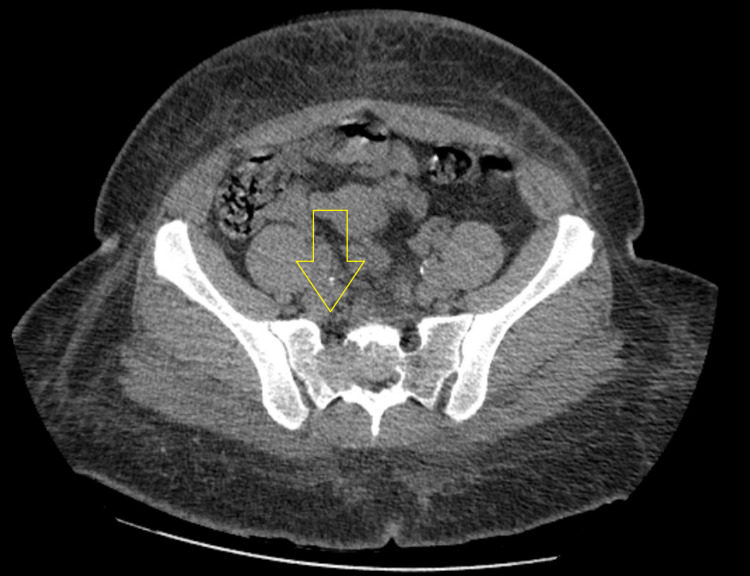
Computed tomography of the pelvis showing a lytic mass within the right sacrum with involved sacral neural foramen and anterior cortical erosion.
